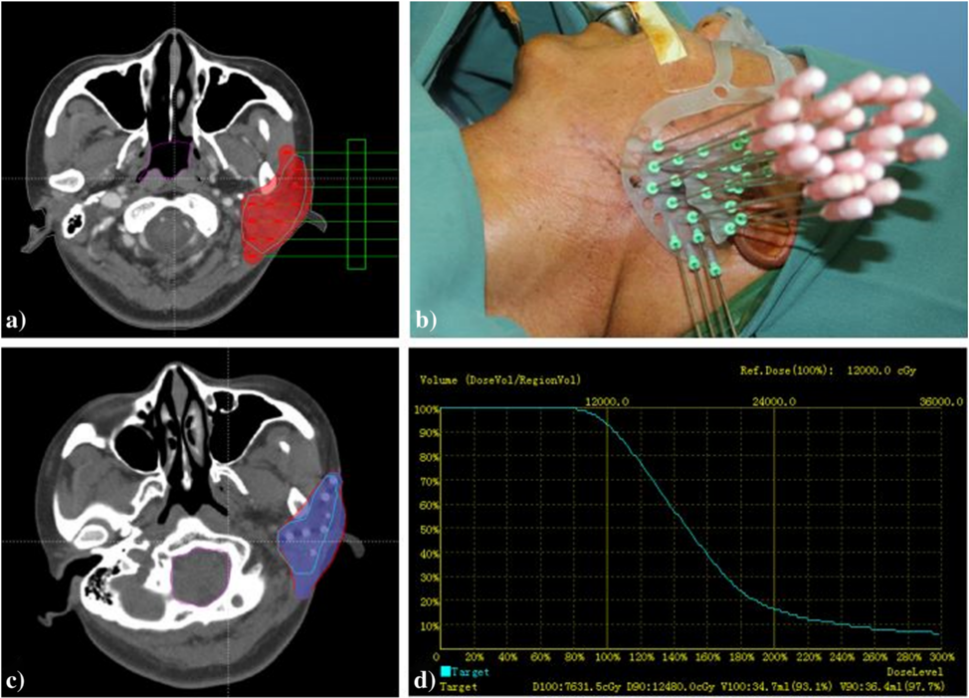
The administration of ^125^I seed parotid brachytherapy. a Isodose curve in the implant plan from CT scan. b Needle implantation c Isodose curve after seed implantation from CT scan. d Dose volume histograms of PTV after seed implantation
